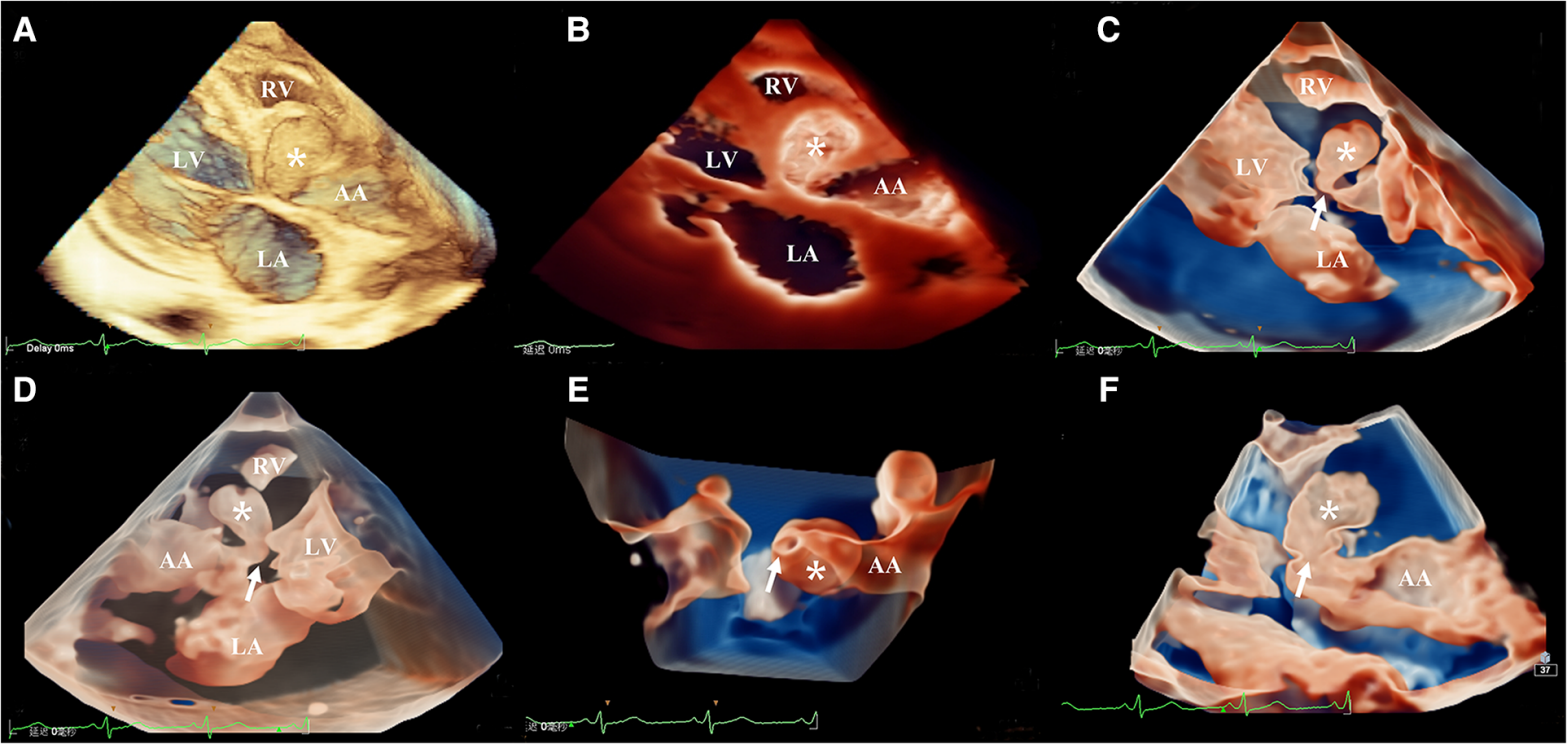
Head-to-head comparisons among traditional three-dimensional transthoracic echocardiography (A), TrueVue (B), and TrueVue Glass (C–F) in the evaluation of the aortic root pseudoaneurysm (white asterisk, its neck: white arrow). Different views of pseudoaneurysm in the TrueVue Glass mode: en face (C), dorsal (D), bottom (E), and internal (F) perspective. TrueVue Glass increased the degree of tissue transparency, providing a translucent view, and allowing one to visualize the full view of pseudoaneurysm, as well as its neck. LA, left atrium; LV, left ventricle; RV, right ventricle; AA ascending aorta.
